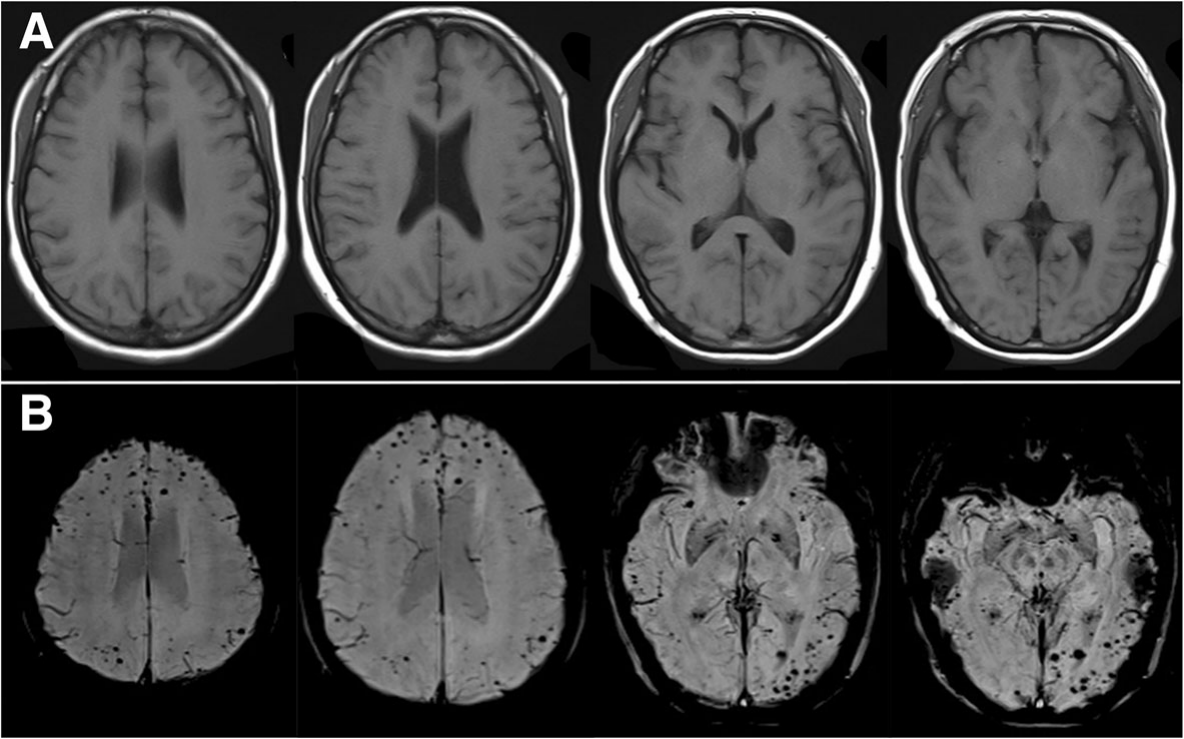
MRI images after the second corticosteroid therapy for four months. a no lesion was found on FLAIR; b microbleeds were not increased on SWI
